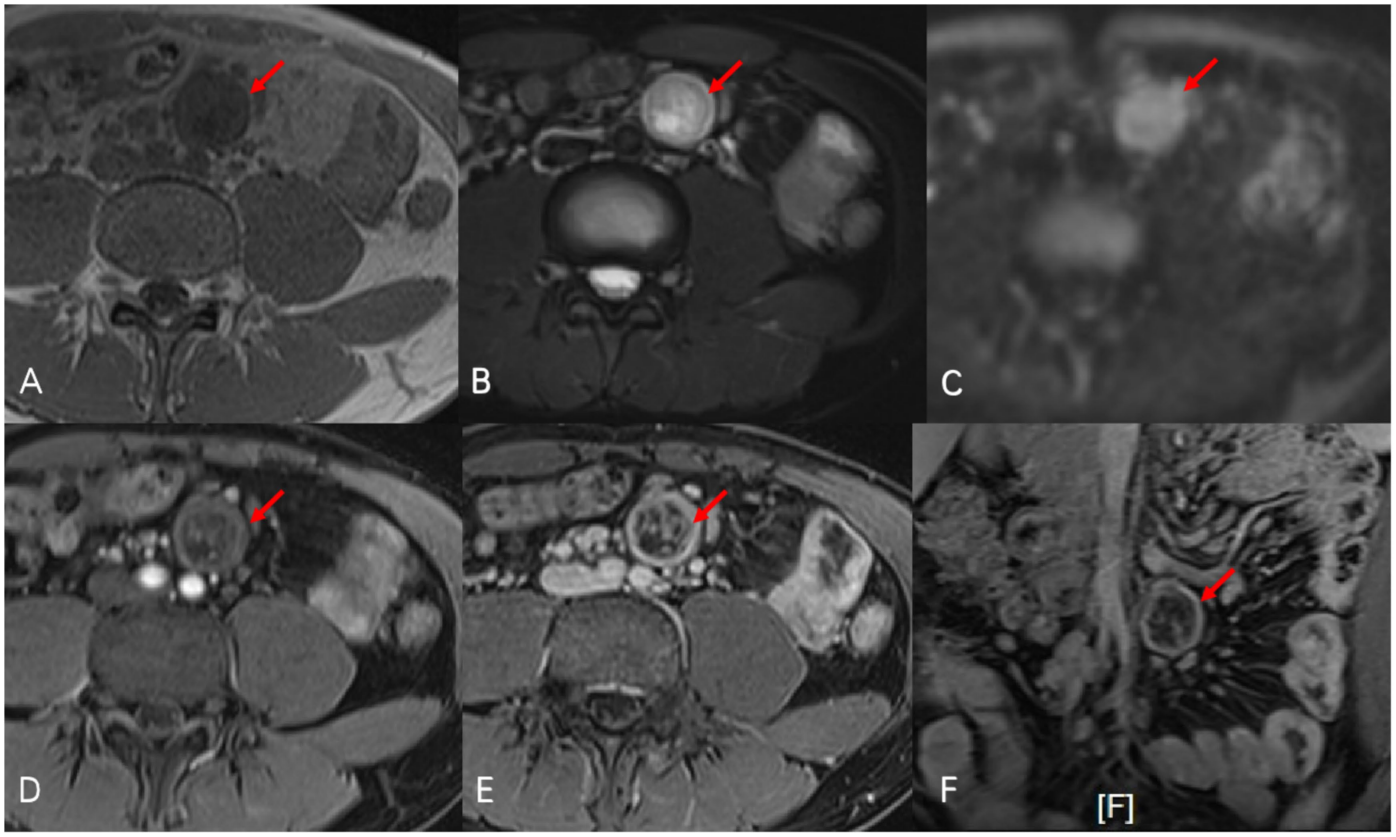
Oval-shaped abnormal signal nodule (red arrow) was observed at the mid-left root of the mesentery in abdominal enhanced MRI. On T1-weighted images (A), the nodule presented as isointense to hypointense, and on fat-suppressed T2-weighted images (B), it showed slightly to markedly higher signals with mixed intensity at the boundaries. The signal is mildly increased on diffusion-weighted imaging (C). After enhancement [(D) arterial phase, (E) portal venous phase, and (F) portal venous phase/coronal], the nodule showed progressive enhancement, with pronounced enhancement at the edges, and a clear cystic change could be seen in the central area.
